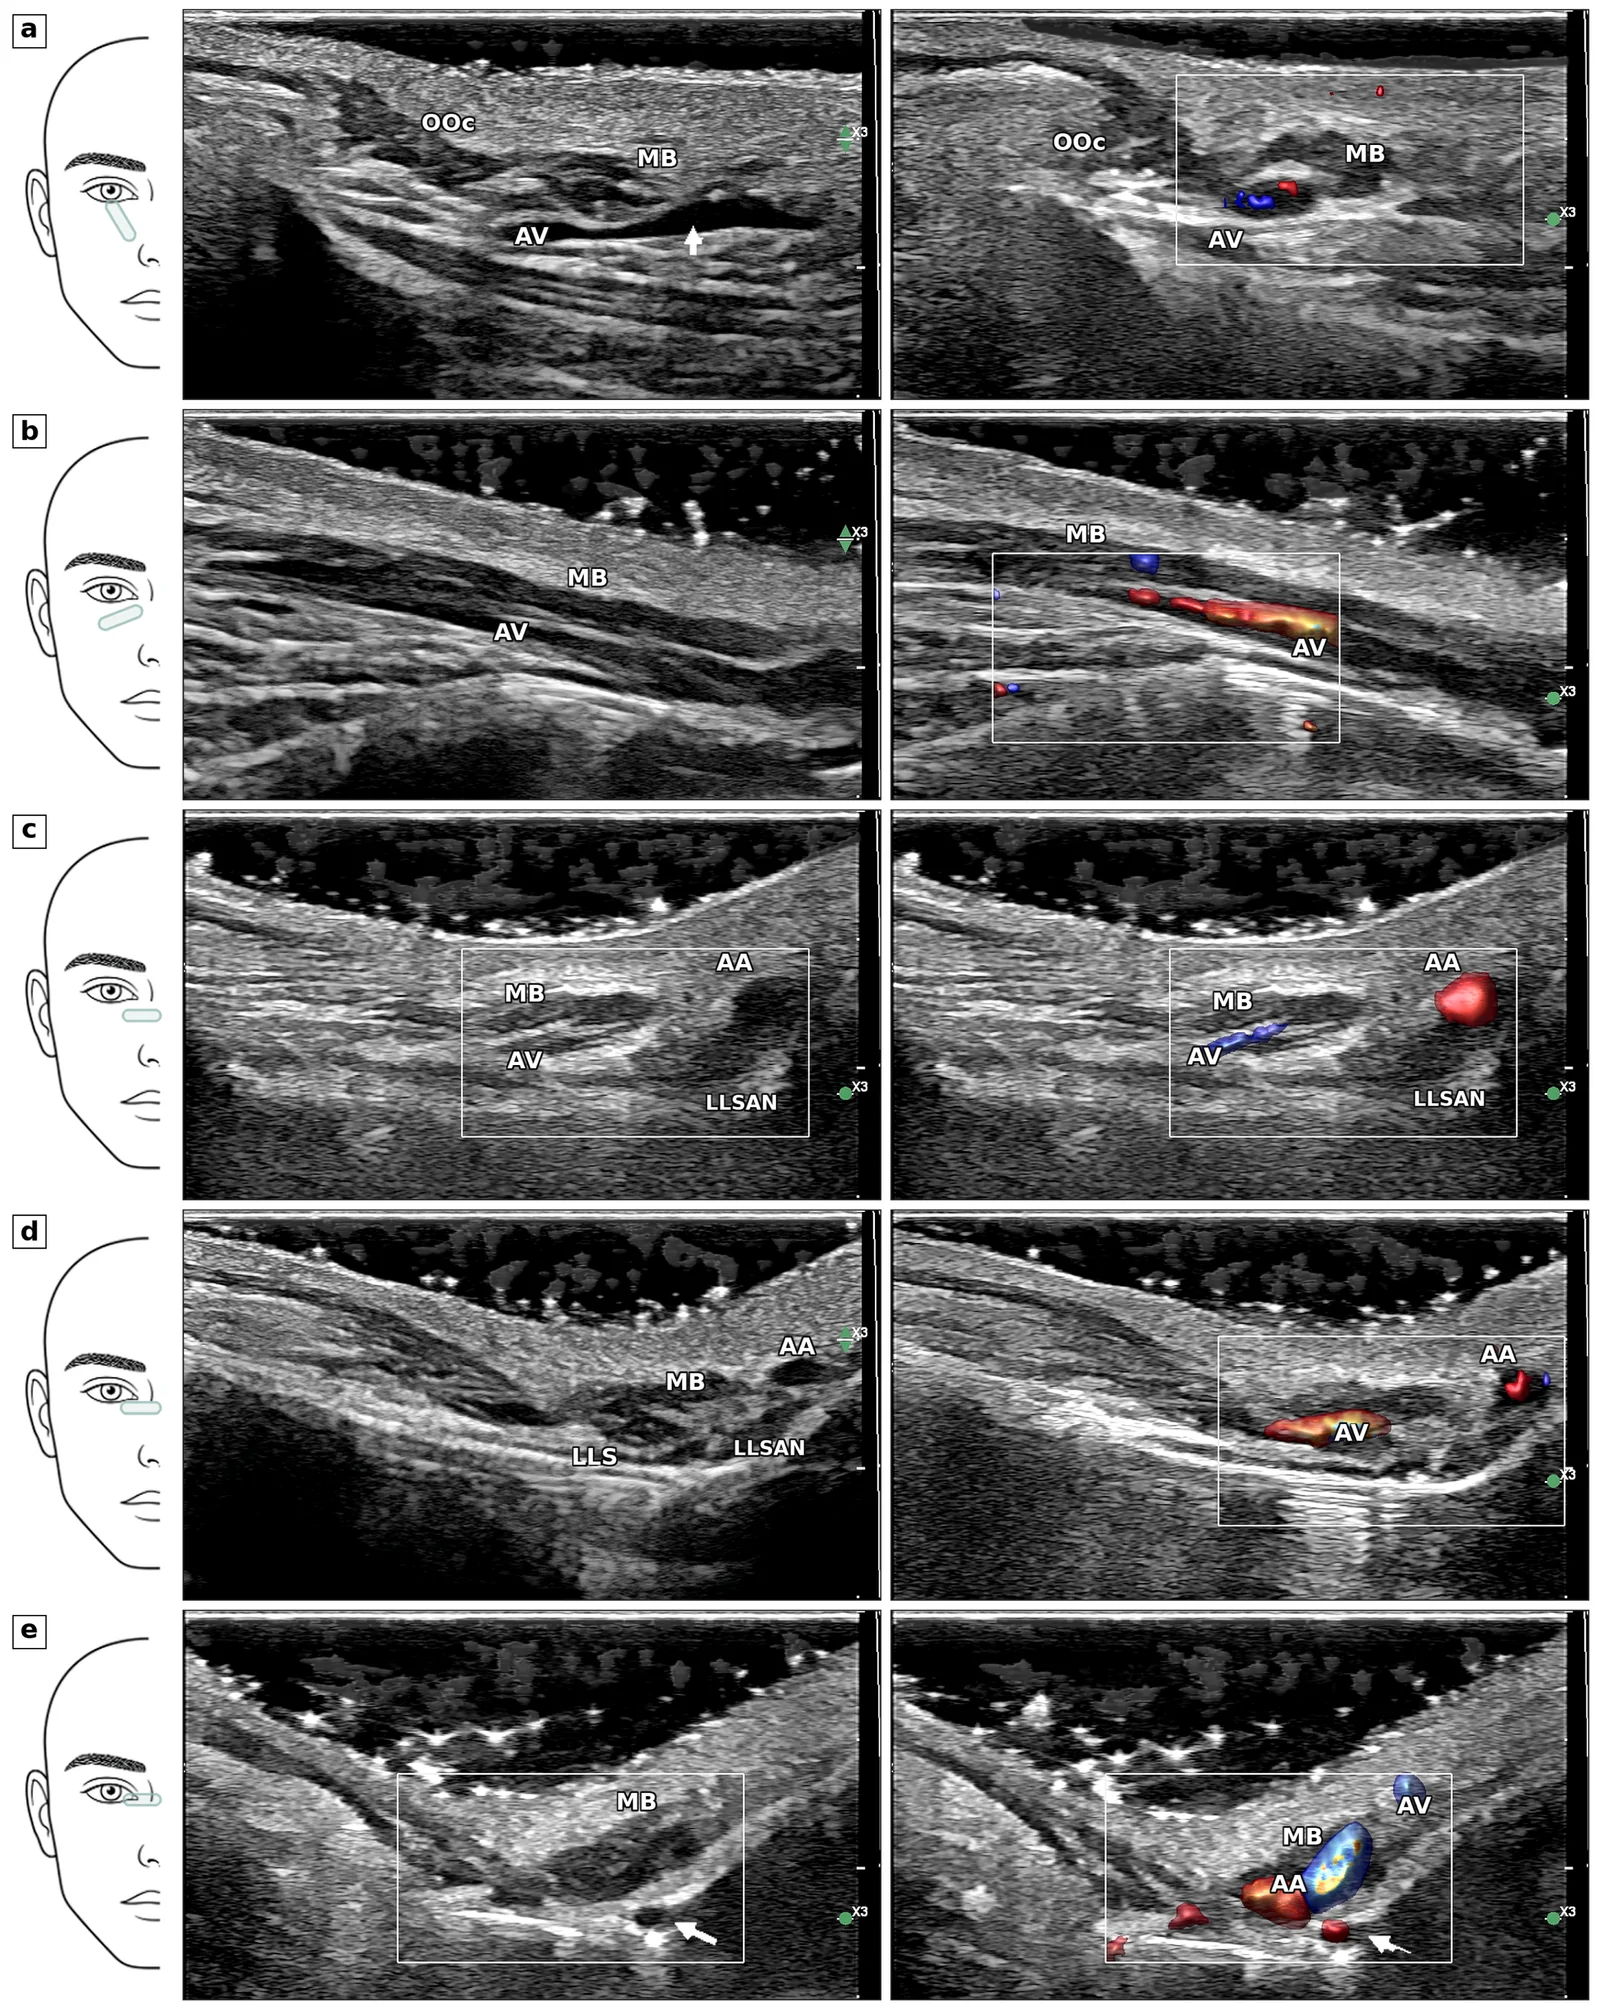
Line-guided high-frequency ultrasound and qualitative color Doppler mapping of the orbicularis oculi (OOc)-related band-like correlate and angular vessels. Ultrasound was performed relative to the clinically marked contour; all panels show the left side. Labels identify the OOc-related band-like correlate, designated MB (medial orbicularis oculi–related band), angular vein (AV), angular artery (AA), and the adjacent levator labii superioris alaeque nasi/levator labii superioris (LLSAN/LLS) field. Probe orientation follows the reference system of Kim et al. [12]; detailed point and line definitions are provided in the Supplementary Technical Note. Where visible, MB lies superficial to the LLSAN/LLS field with an intervening adipose plane. (a) Transverse section near the midpupillary-line reference (PL2). The arrow indicates a superficial venous tributary passing beneath MB; this relationship is further shown in Supplementary Figure S1 and correlated on CT in Figure 6. (b) Longitudinal section along MB between P6 and P7; AV lies deeper. (c–e) Consecutive transverse views proceeding superiorly toward the frontal process of the maxilla and medial tear trough. Arrows indicate bony canal openings and emerging vessels associated with AA; in panel (e), the arrow indicates a bony canal and a vessel emerging from its opening. Ultrasound showed more small bony foramina/canal openings along the examined course than were apparent on the corresponding CT images.
